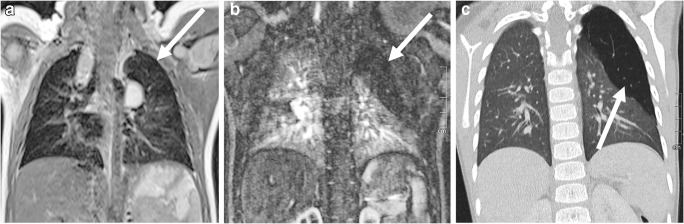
Emphysema of the left upper lobe in a 7-day-old boy on lung MRI (parameters in Table 2). a The upper-lobe emphysema is not clearly visible on coronal T2-W turbo spin-echo image (*arrow*). It is caused by a small single thoracic cyst with a diameter of 2 cm. b The over-inflation with the resulting capillary reduction (*arrow*) only becomes visible on the time-resolved coronal MR angiogram because of the lack of contrast enhancement of the left upper lobe. c Corresponding coronal CT image shows the left upper lobe over-inflation (*arrow*)
